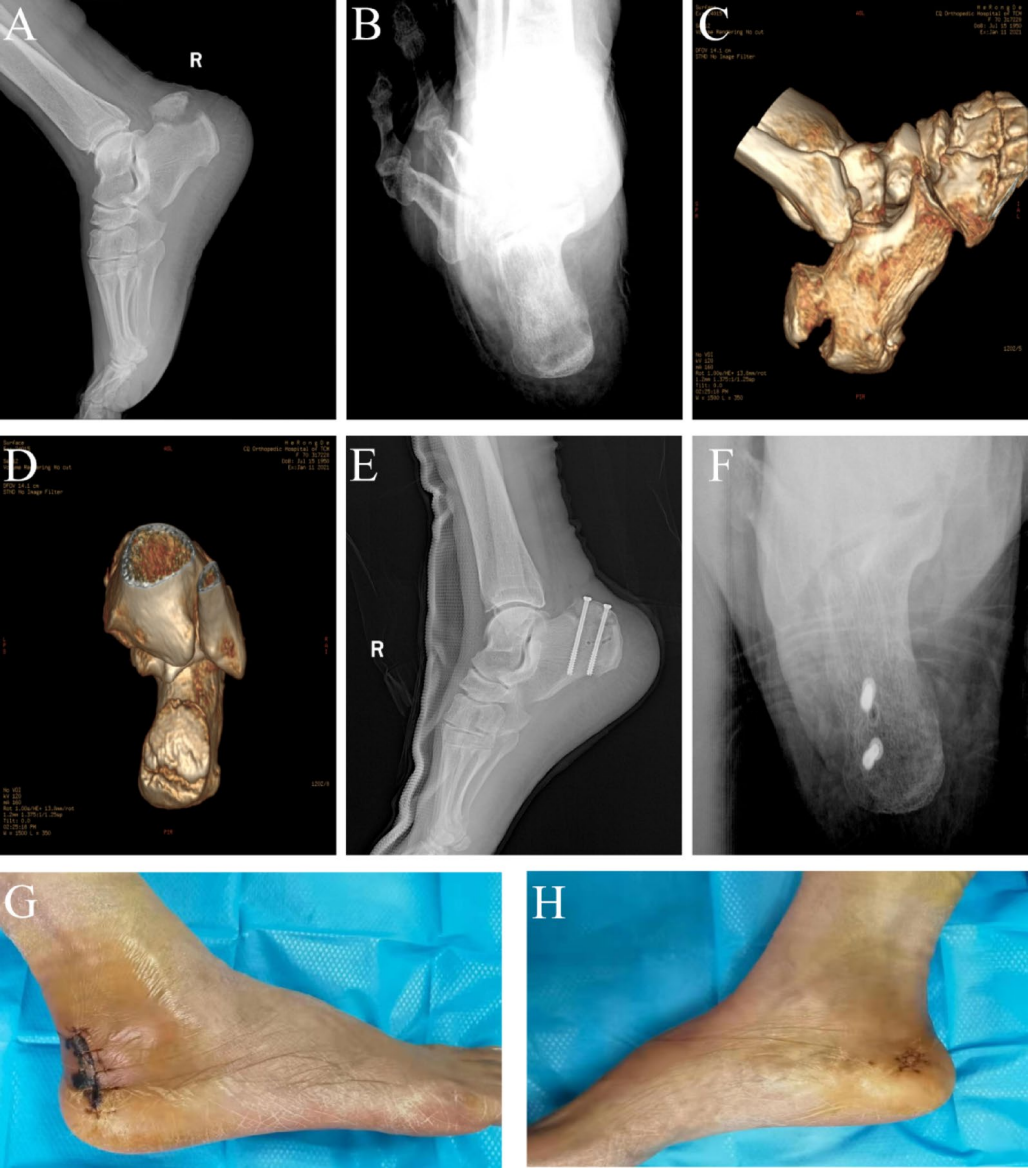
Preoperative imaging and postoperative outcomes in a representative case of Beavis Type II posterior calcaneal tuberosity avulsion fracture. A–B: Preoperative dorsoplantar and lateral radiographs; C–D: Preoperative axial and sagittal CT reconstructions; E–F: 3-month postoperative radiographs demonstrating fracture union; G–H: Wound appearance at 2-week suture removal
